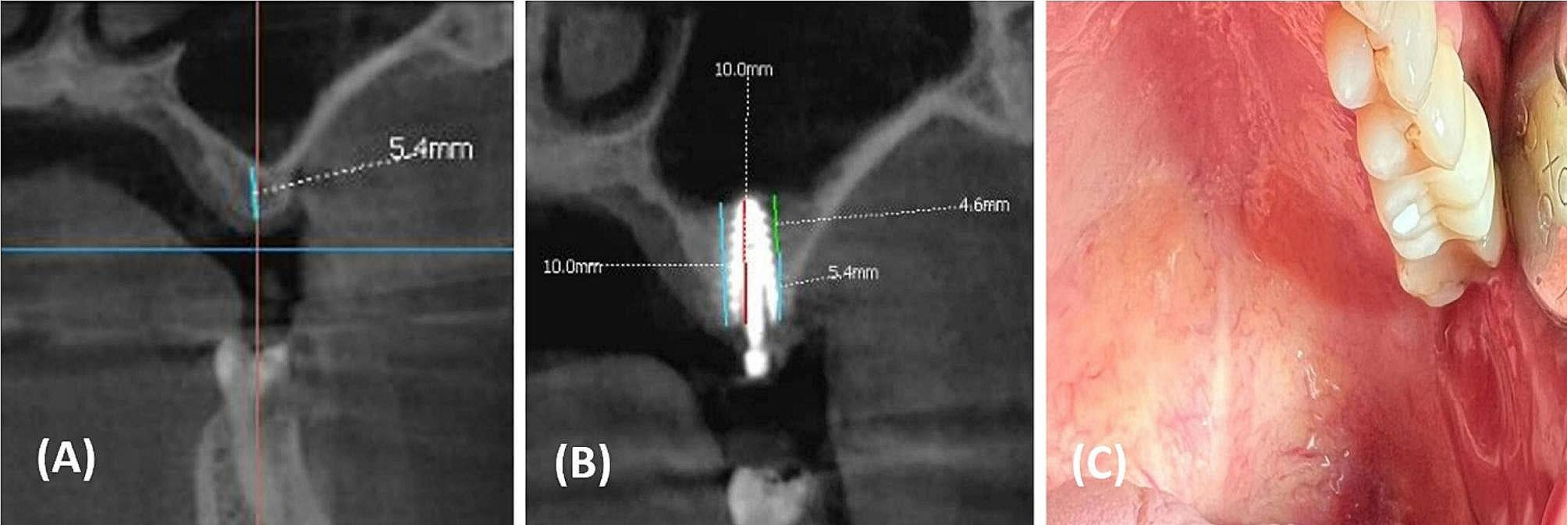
Preoperative and postoperative CBCT scan views of case with healed socket who needed dental implant in the posterior maxilla and was diagnosed with a reduced vertical bone height the maxillary sinus floor at left upper sex molar; (A) Preoperative coronal view of left upper sex molar, (B) Postoperative coronal view of left upper sex molar at one year after maxillary sinus lifting and implant insertion, (C) Postoperative clinical view
